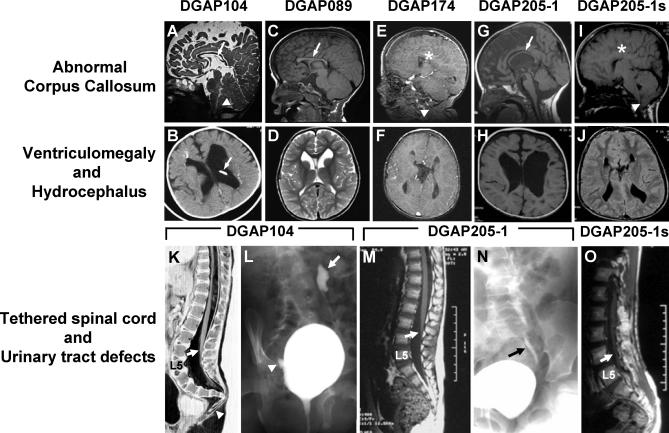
CNS and Urinary Tract Defects in Five Individuals with 1p31.3 Rearrangements (A–J) Brain CT or MRI show a thin corpus callosum in DGAP104 (arrow in A), DGAP089 (arrow in C), and DGAP205–1 (arrow in G); and agenesis of the corpus callosum in DGAP174 (asterisk in E) and DGAP205-1s (asterisk in I). Chiari type I malformation, a downward displacement of the tip of the cerebellar tonsils below the foramen magnum, was found in DGAP104 (arrowhead in A), DGAP174 (arrowhead in E), and DGAP205-1s (arrowhead in I). Congenital ventriculomegaly is present in DGAP089 (D), DGAP174 (F), and DGAP205-1s (J), and hydrocephalus was found in DGAP104 (B) and DGAP205–1 (H). An occipital shunt (arrow in B) was placed in DGAP104 to relieve severe hydrocephalus. (K) DGAP104 MRI shows a tethered spinal cord, with the extremity of the conus medullaris (arrow) at the level of the L4 vertebral body. Arrowhead shows a fishhook deformity of the lower sacral and coccygeal vertebrae. (L) VCUG of DGAP104 depicts left vesicoureteral reflux with retrograde tracking of dye through the ureter into the renal pelvis (arrow) and a right diverticulum at the ureterovesical junction (arrowhead). (M) Spine MRI of DGAP205–1 shows a tethered spinal cord with conus lying at the L3/L4 level (arrow). (N) VCUG of DGAP205–1 shows left vesicoureteral reflux (arrow). (O) Spine MRI of DGAP205-1s depicts a tethered spinal cord with conus lying at L5 (arrow).
